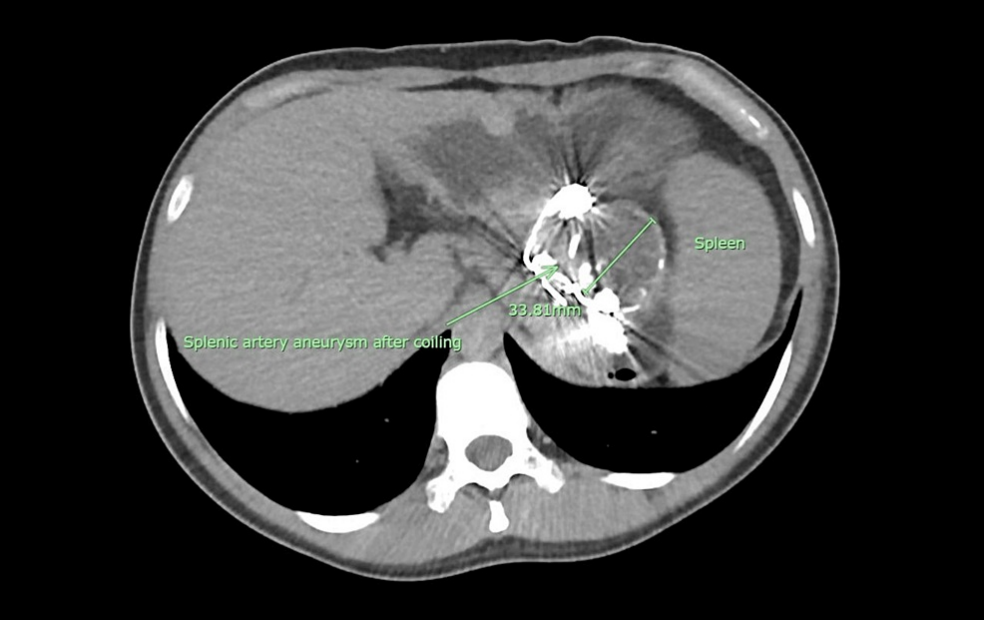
Computed tomography (CT) scan depicting thrombosed splenic artery aneurysm (SAA) with coils within
